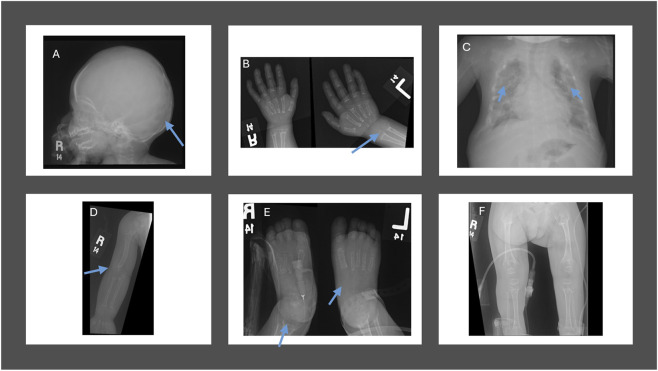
Skeletal survey at 1 years of age in an infantile hypophosphatasia case (Case 15). (A) Skull x-ray showed poor ossification of the calvarium, especially the occipital and parietal bone, with generalized osteopenia. (B) Hand radiograph revealing multiple bony lucencies, broadening of the metaphysis, and delayed ossification centers. (C) Thin osteopenic ribs are noted, associated with bilateral multiple fractures. (D) Cannot distinguish the margins of the metaphysis, demonstrating multiple bony lucencies and widened metaphysis, indicative of defective mineralization. (E) Osteopenia in bilateral feet with fraying of the distal tibia and fibula. (F) Increase in distance of the metaphyseal ends. Metaphyseal flaring of the knees. Convexity of the distal metaphysis of the femur. Faint periosteal reactions in the femur.
